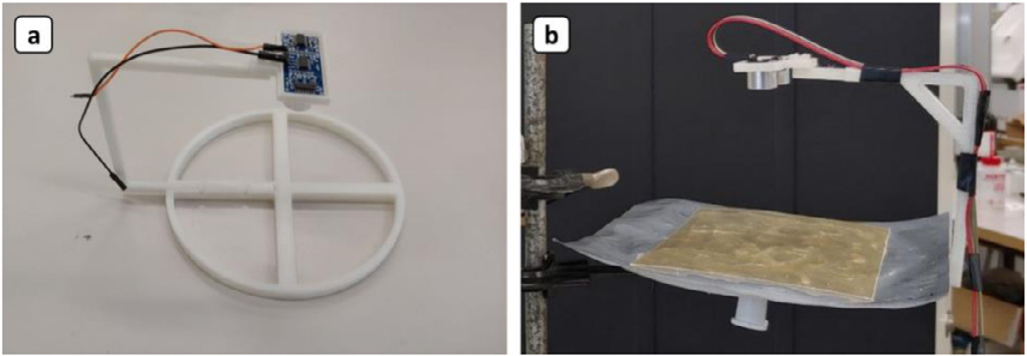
(a) 3D-Printed PLA structure assembled with HC-SR04™ Ultrasonic sensor. (b) Final structure of the Digital Gasometer with a Supel™ 30,226-U gasbag and a rectangular plastic piece (yellow) to homogenize the surface (right).
